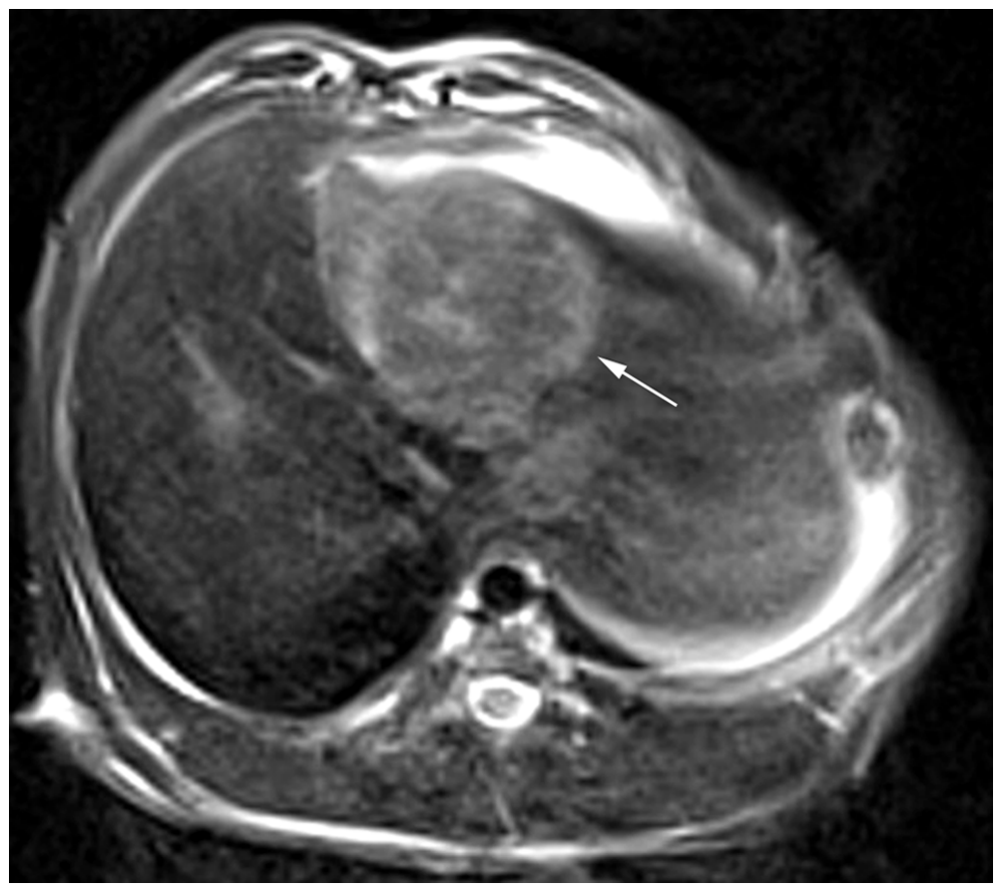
MR image of a VX2 tumor. T2-weighted MR image shows a hyper-intensity VX2 tumor in the left liver lobe (arrow).
